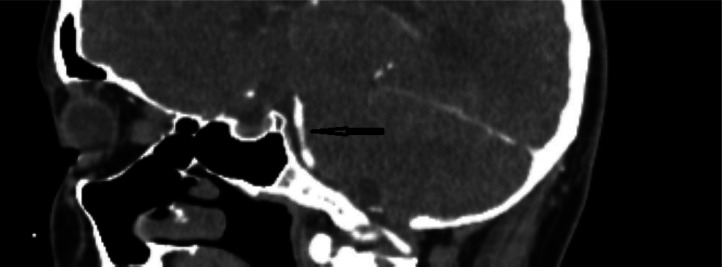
CTA sagittal section showing midbasilar stenosis due to dissection.
